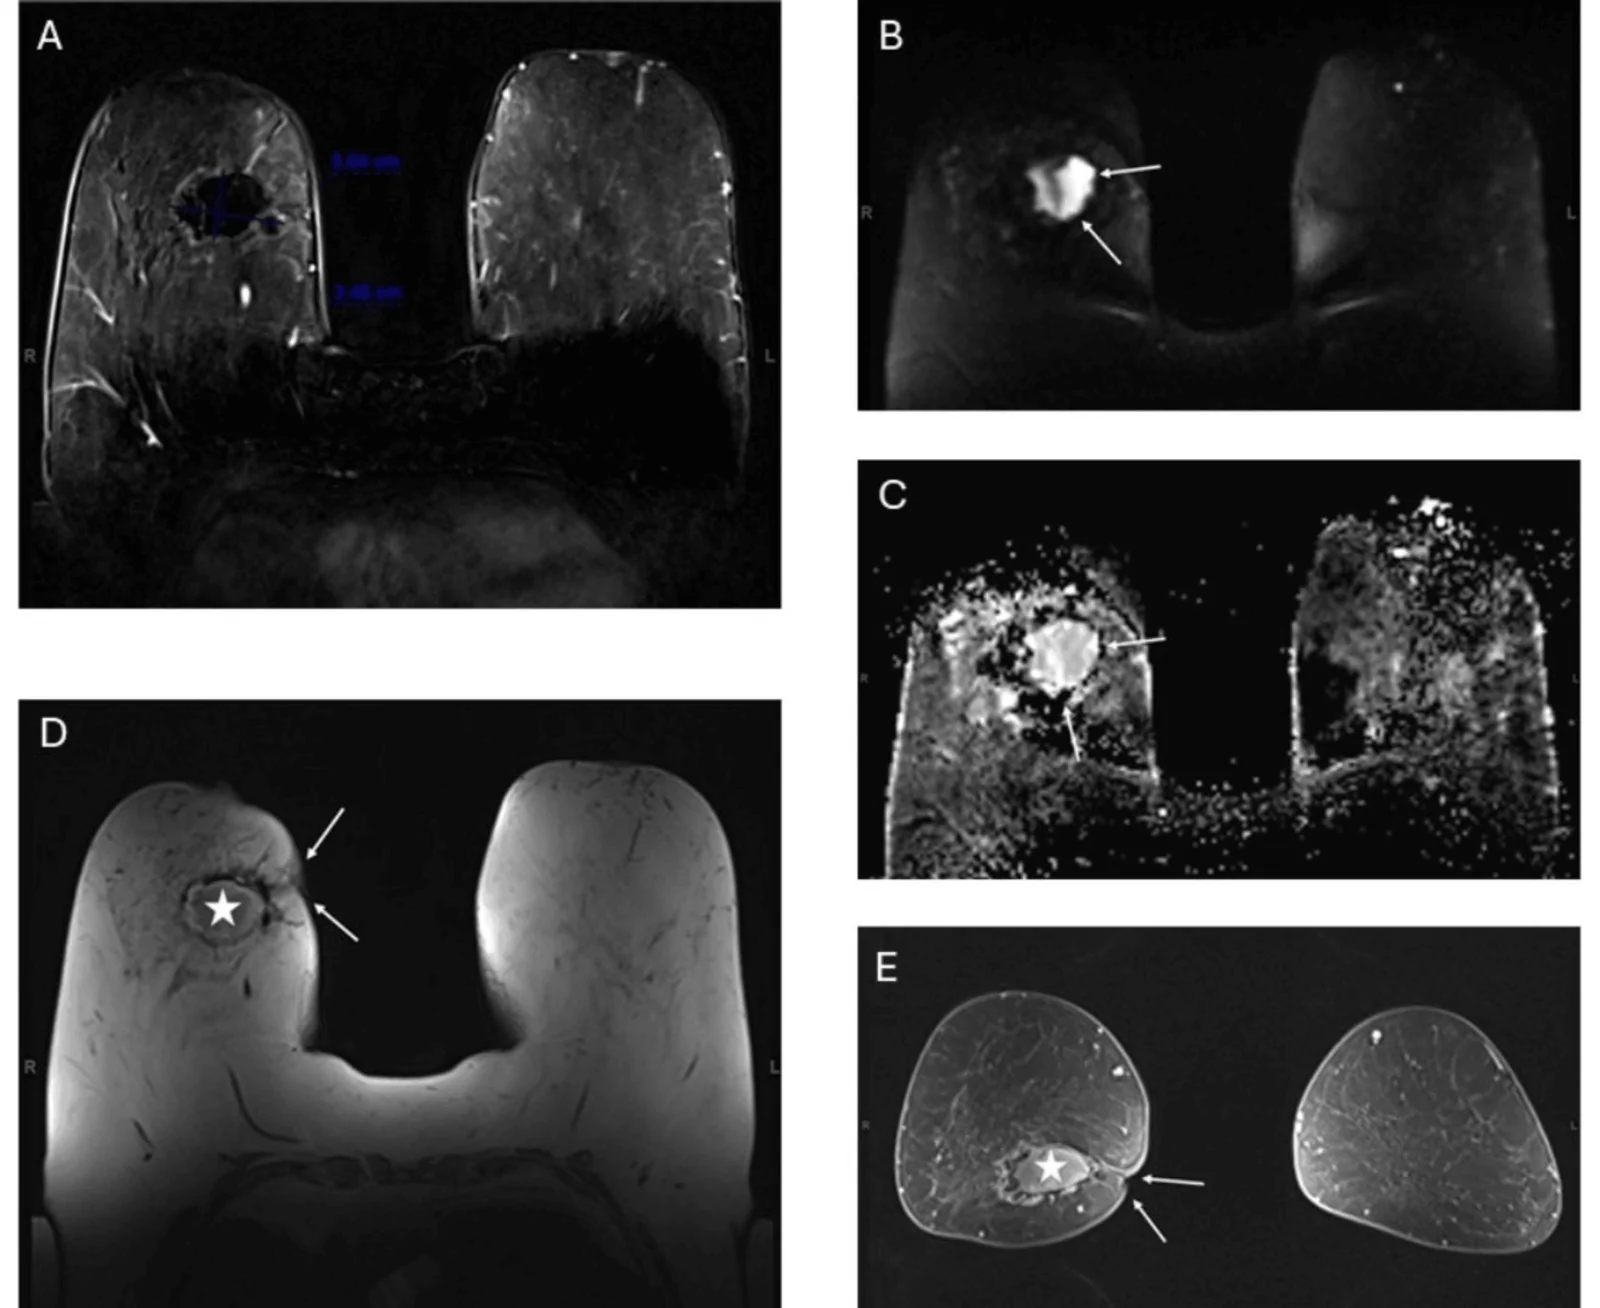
Breast magnetic resonance imaging showing a multicompartmental T1-hyperintense mass (stars, D–E) measuring 4.6 × 3.5 × 4.9 cm in the inferomedial right breast with subtle peripheral enhancement (measurements, A), no diffusion restriction (arrows, B–C), and associated skin retraction (arrows, D–E) (BI-RADS IV).
